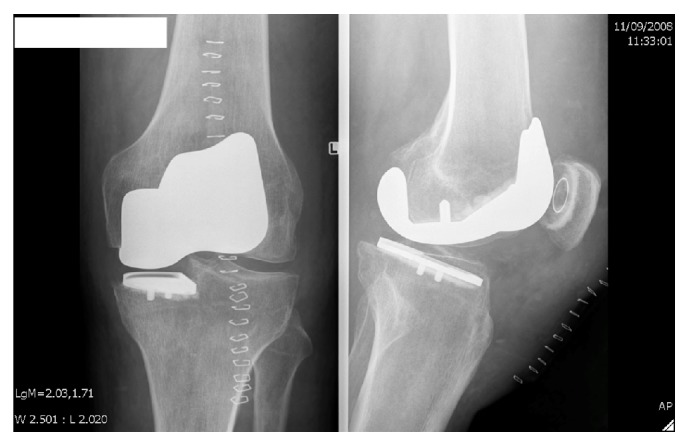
Anteroposterior and profile view X-rays of the knee following surgery.
